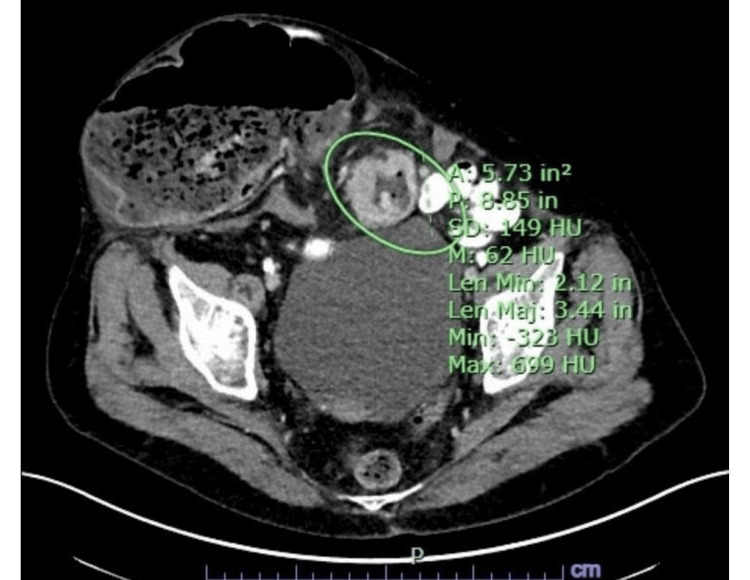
Axial CT scan showing distended cecum and air-fluid levels, indicating significant bowel obstruction
